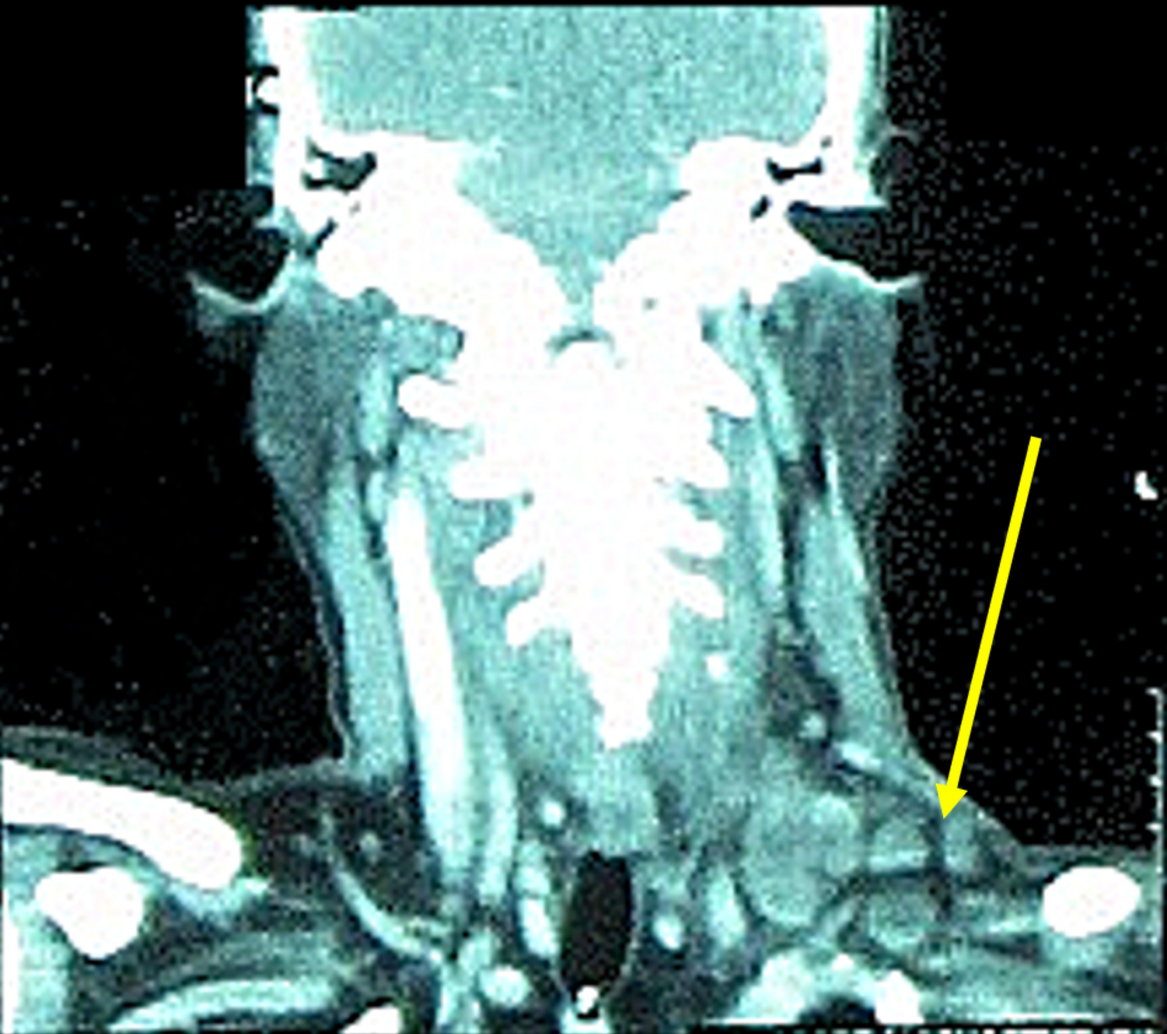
Coronal CT scan of the neck showing cervical lymphadenopathy due to metastatic disease
